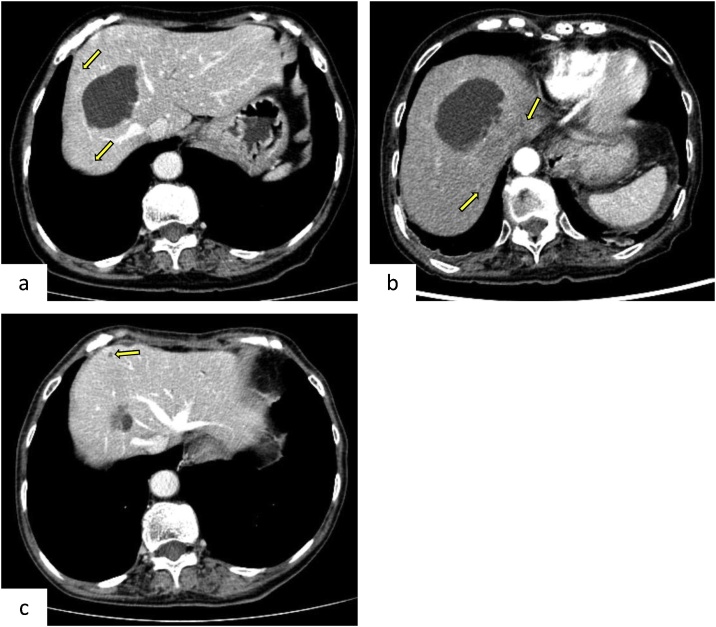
Contrast-enhanced abdominal computed tomography (CT). The arrow indicates the asymptomatic cyst. a. Preoperative CT shows a hepatic cyst, measuring 6.0 × 3.0 cm, in segment VIII. b. The border of the hepatic cyst is slightly enhanced on CT at 3 days after readmission. c. Repeated sclerotherapy using minocycline hydrochloride decreases the infected hepatic cyst from 6.0 to 1.7 cm.
